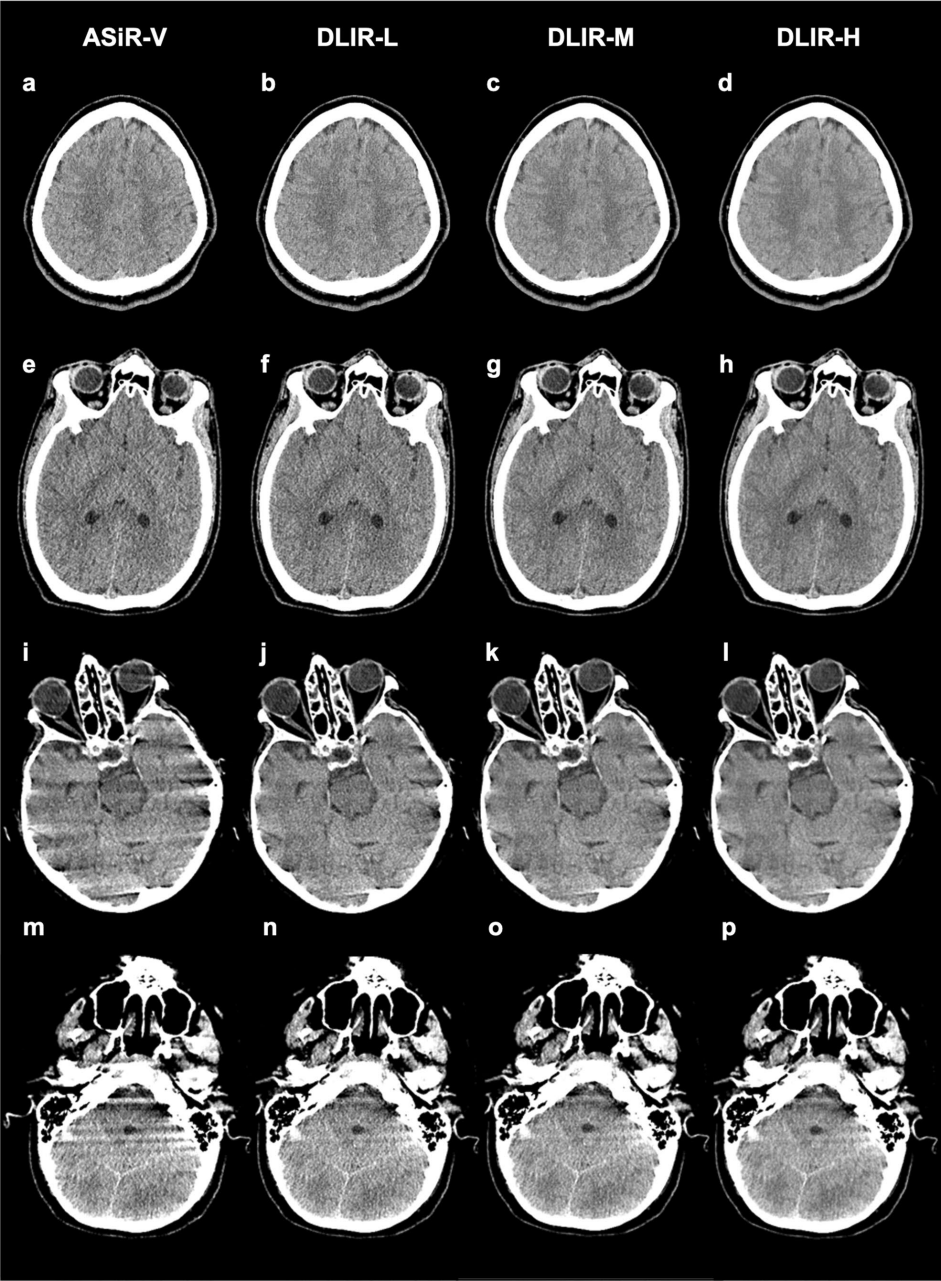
Axial noncontrast trauma head CT images reconstructed with ASiR-V (a, e, i, m), DLIR-L (b, f, j, n), DLIR-M (c, g, k, o), and DLIR-H (d, h, l, p). The first row of images (a–d) is of a 24-year-old male patient at the level of the centrum semiovale. The second row of images (e–h) is of a 41-year-old male patient at the level of the basal ganglia. The third row of images (i–l) is of a 67-year-old female at the level of the pons/interpeduncular cistern. The fourth row of images (m–p) is of a 36-year-old female at the level of the pons/interpetrous region. Readers graded image noise, brain structures, and artifacts higher for the DLIR reconstructions compared to ASiR-V. In addition, there was a tendency towards higher scores with increasing DLIR strength levels
